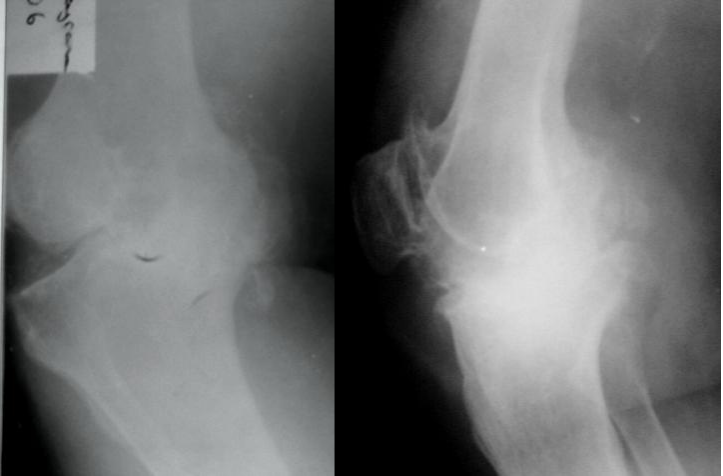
AP and lateral roentgenography of the knee before the first operation.
